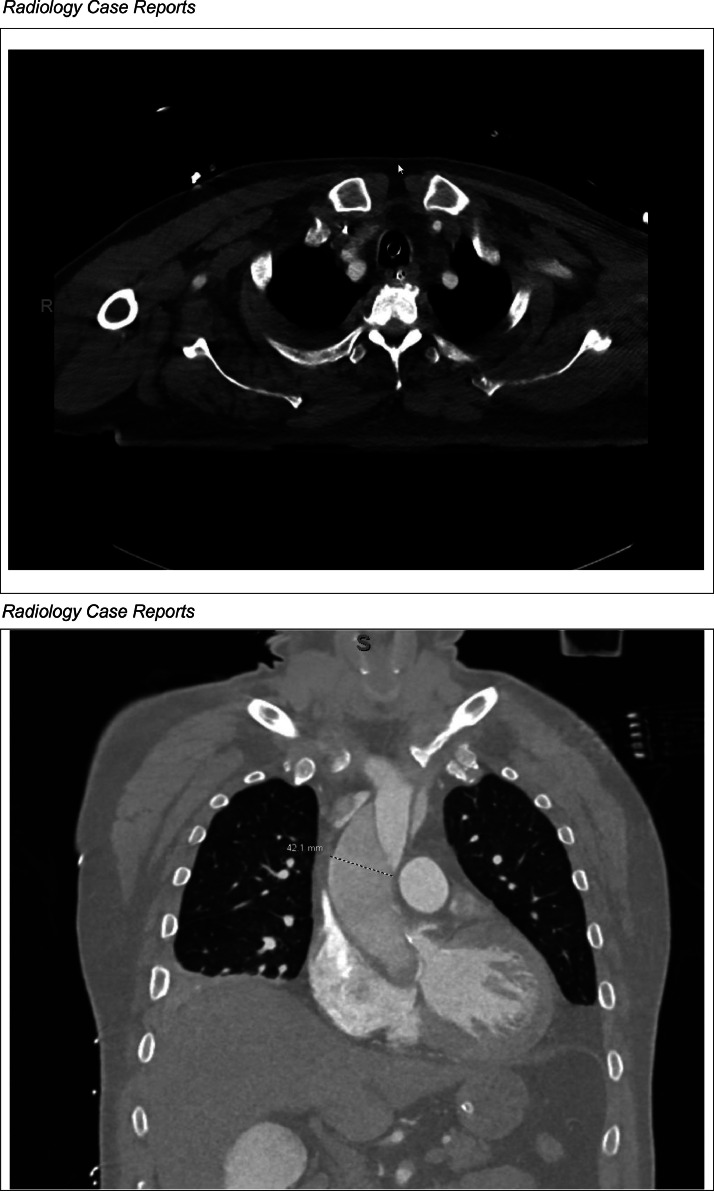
Computed tomographic angiography demonstrating 42.1 mm diameter of the mid-ascending aorta with dissection flaps.
